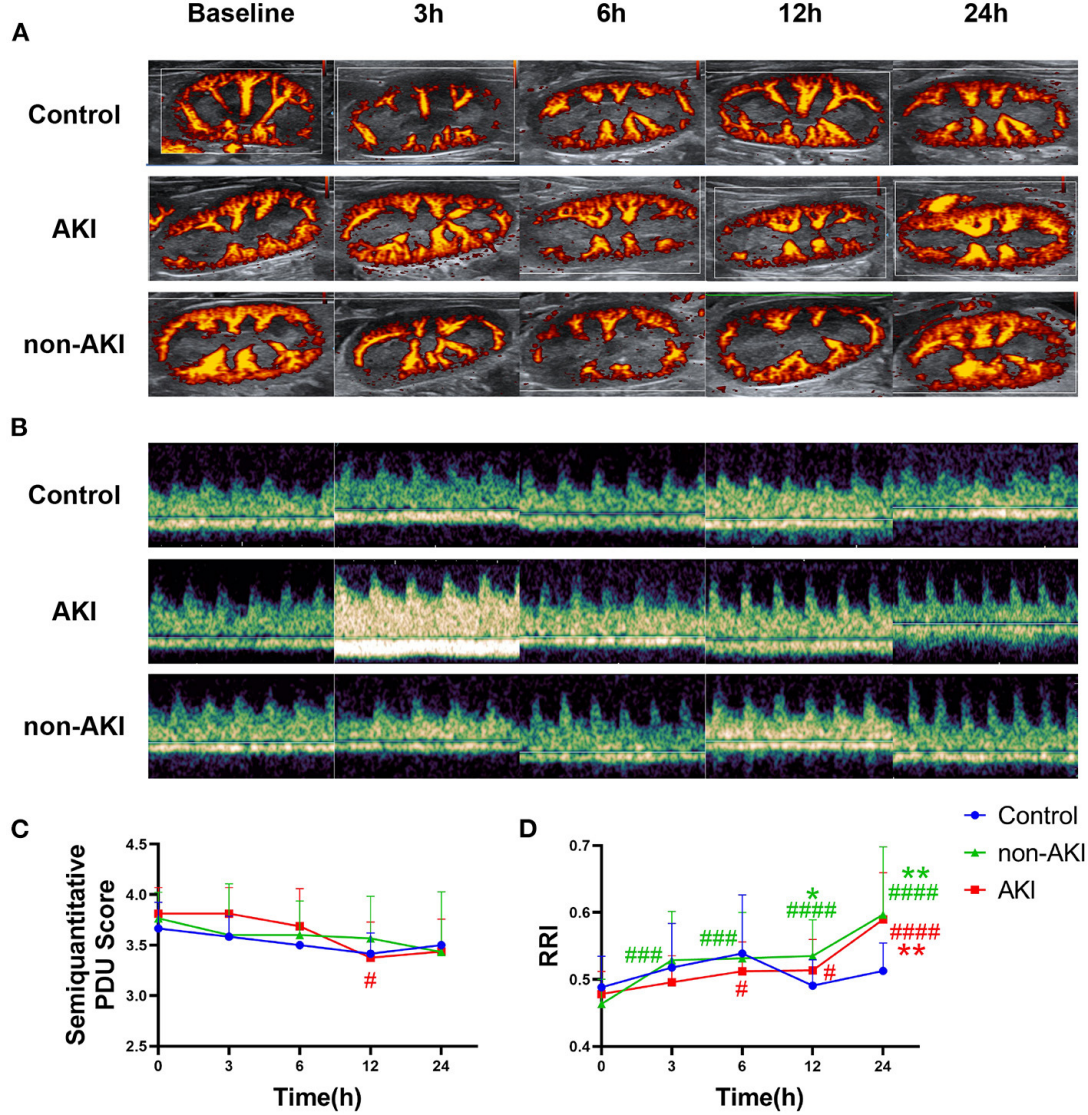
Changes in renal ultrasonography during the observation from baseline to 24 h after operation. (A) Example images showing the renal blood flow using power Doppler ultrasound (PDU) in control, AKI, and non-AKI groups at baseline, 3, 6, 12, and 24 h. (B) Example images showing the renal resistive index (RRI) using pulsed wave Doppler in control, AKI, and non-AKI groups at baseline, 3, 6, 12, and 24 h. (C) Changes of semi-quantitative PDU score over time. Changes in renal blood flow were relatively mild in each group, with only the AKI group showing a statistically significant decrease at 12 h from baseline. (D) Changes of RRI over time. RRI increased gradually in both AKI and non-AKI groups. Compared with baseline, a significant increase in RRI first appeared at 3 h in non-AKI group and at 6 h in the AKI group. Compared with the control group, the RRI of non-AKI group was significantly higher at 12 and 24 h, while significantly higher only at 24 h in the AKI group. There was no significant difference in RRI between the AKI group and the non-AKI group at each time point. Data are expressed as mean ± SD. Using two-way repeated-measures ANOVA with the Geisser–Greenhouse correction, followed by Tukey's multiple comparisons test or Sidak's multiple comparisons test, *P*-values are shown. P* < 0.05, *P* < 0.01, compared to control group; #*P* < 0.05, ###*P* < 0.001, ####*P* < 0.0001, compared to baseline. AKI, acute kidney injury; PDU, power Doppler ultrasound; RRI, renal resistive index.
